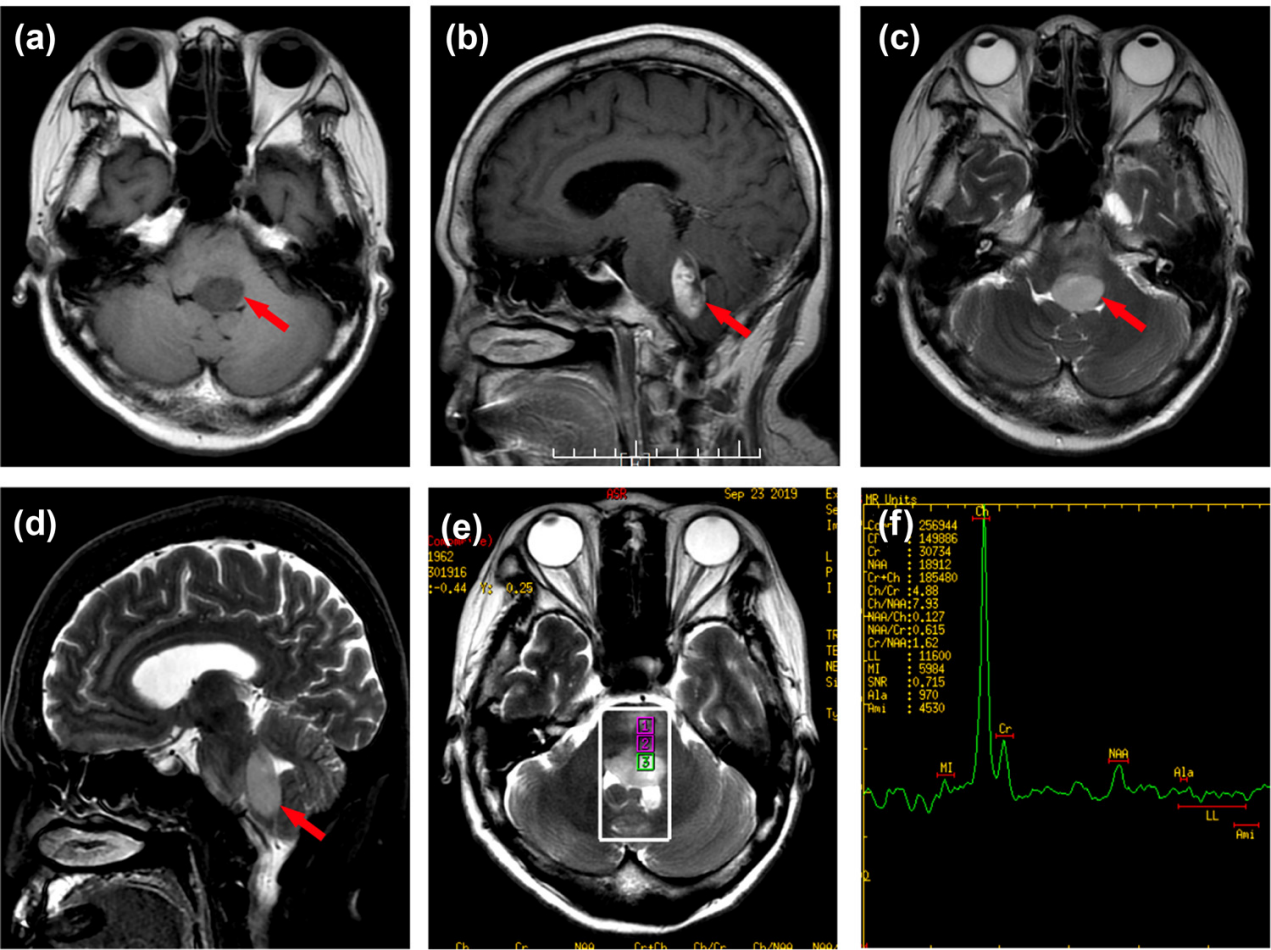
MRI performed after 4 weeks. (a) T1WI revealed that the further enlargement of abnormal low signal intensity (arrow). (b) T1-FLAIR, (c) T2WI, and (d) T2-FLAIR revealed that the further enlargement of abnormal high signal intensity in the brainstem and the compression on the surrounding brain tissue was more obvious (arrows). (e and f) Cho/NAA = 7.93, Cho/Cr = 4.88, NAA/Cr = 1.21 of MRS point of interest 3.
